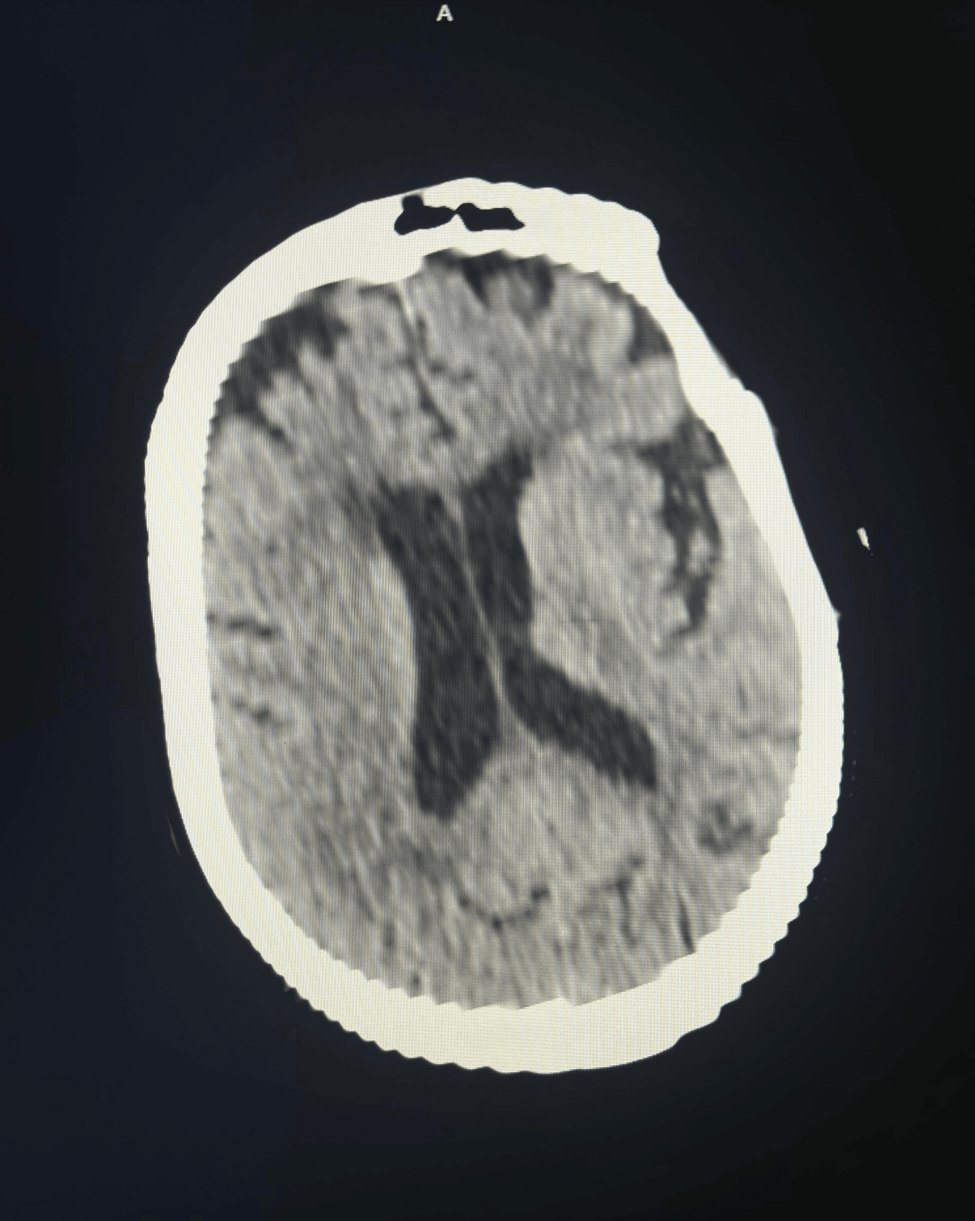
Head computed tomography cross-sectional image shows age-related degenerative changes and mild brain atrophy, otherwise unremarkable for any other pathological findings.
